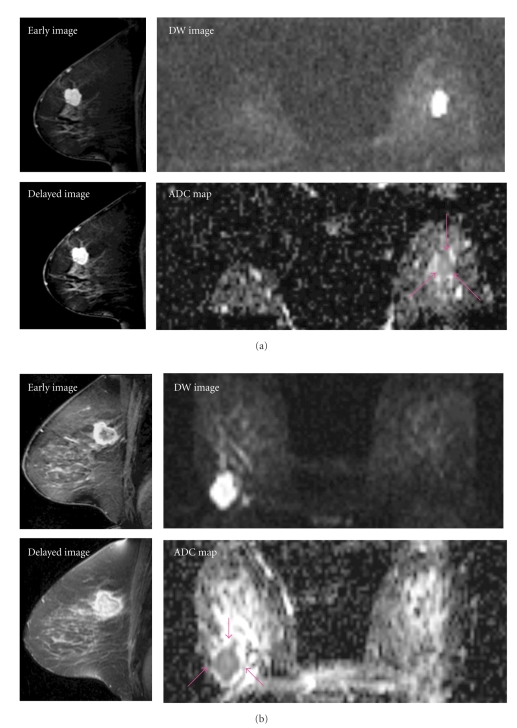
MR images of representative cases. (a) Case 3: a 59-year-old woman with invasive carcinoma of the left breast. E/D ratio: 1.03, ADC value 0.850 × 10^−3^ mm^2^/s. A well-demarcated mass with strong enhancement is observed. The mass shows high intensity on DWI (b = 1000). (b) Case 13: a 72-year-old woman with invasive carcinoma in the right breast. E/D ratio: 0.90, ADC value 0.975 × 10^−3^ mm^2^/s. A mass with early rim enhancement with delayed internal enhancement is shown. The mass shows high intensity on DWI (b = 1000). The central portion shows relatively low signal intensity than that in the periphery. The central area showing delayed enhancement may have abundant fibrous stroma.
